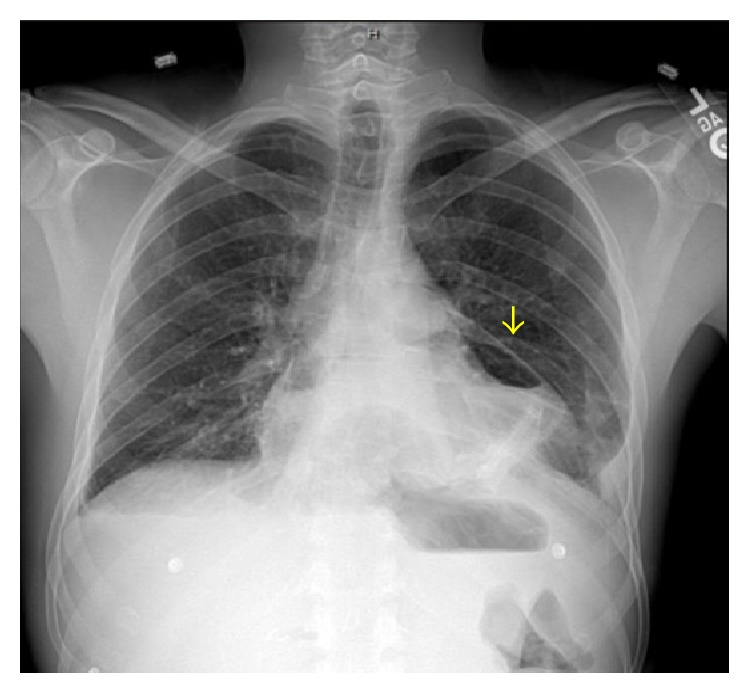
PA chest X-ray. A halo is visible around the left cardiac border.
